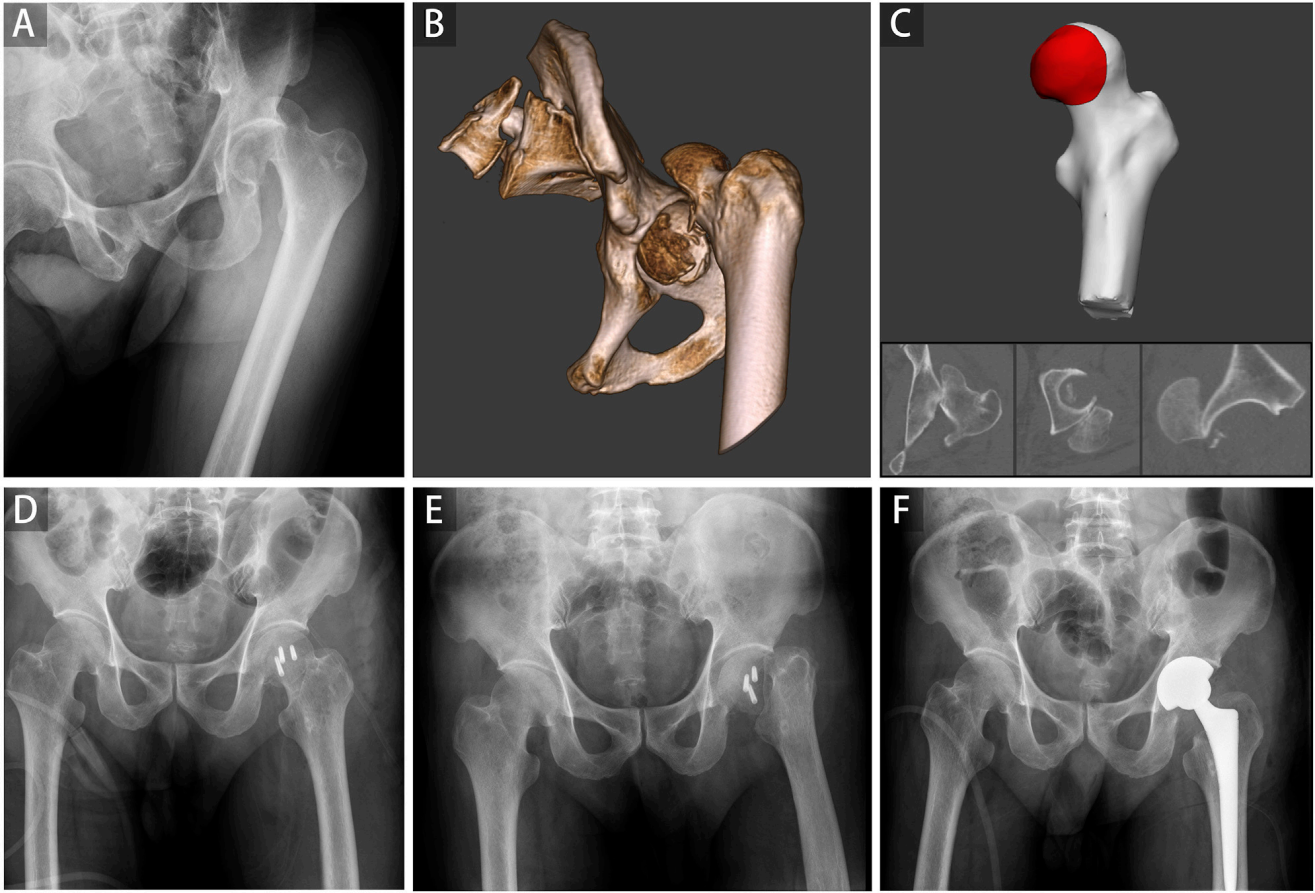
A representative case of the refracture of the femoral neck after internal fixation of the femoral head fracture was shown. A 62-year-old man involved in a severe traffic accident was diagnosed with femoral head fracture based on X-ray (A) and CT (B). The average percentage of maximum defect length across three planes of 96.64% in the 2D injury model and the percentage of fracture area of 40.72% in the 3D injury model were determined (C). Open reduction and internal fixation were conducted, and the postoperative radiograph showed good reduction status (D). A refracture of the femoral neck occurred without trauma and fall 3 weeks postoperatively (E). Finally, hip replacement surgery was performed (F).
